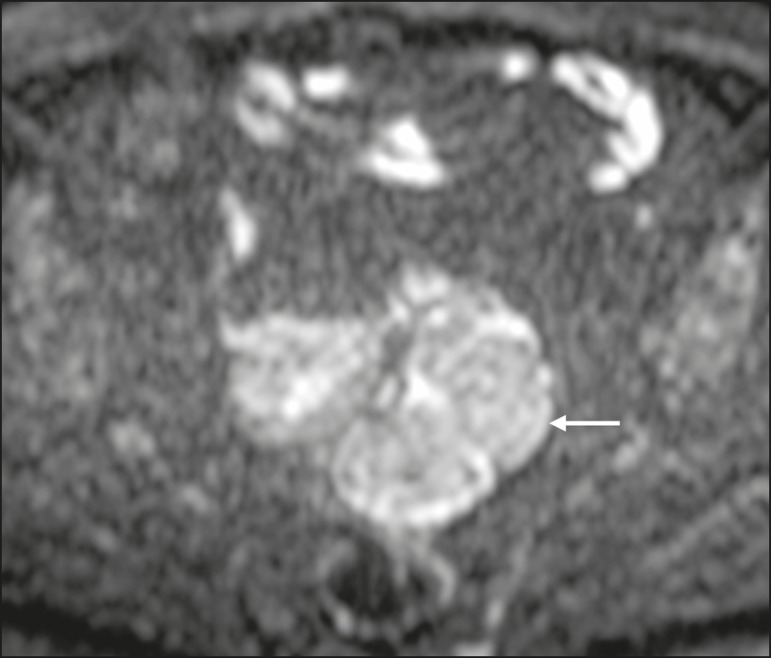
MRI of a female patient with fibroma. Axial DWI showing that the tumor
